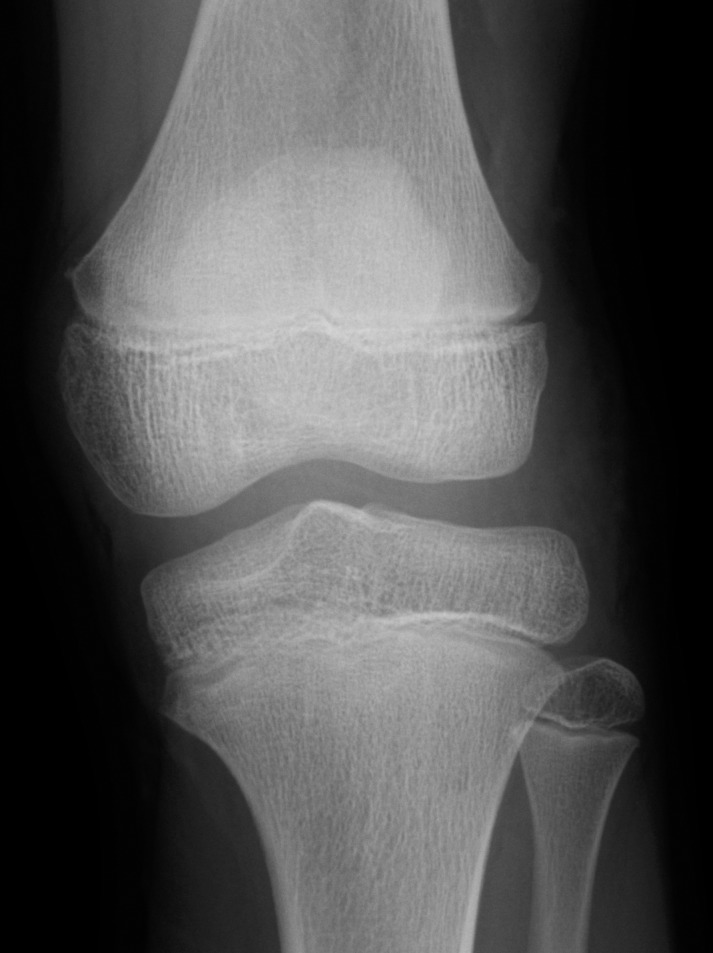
Plain radiographs of the knee joint did not show calcified and osseous lesions.
